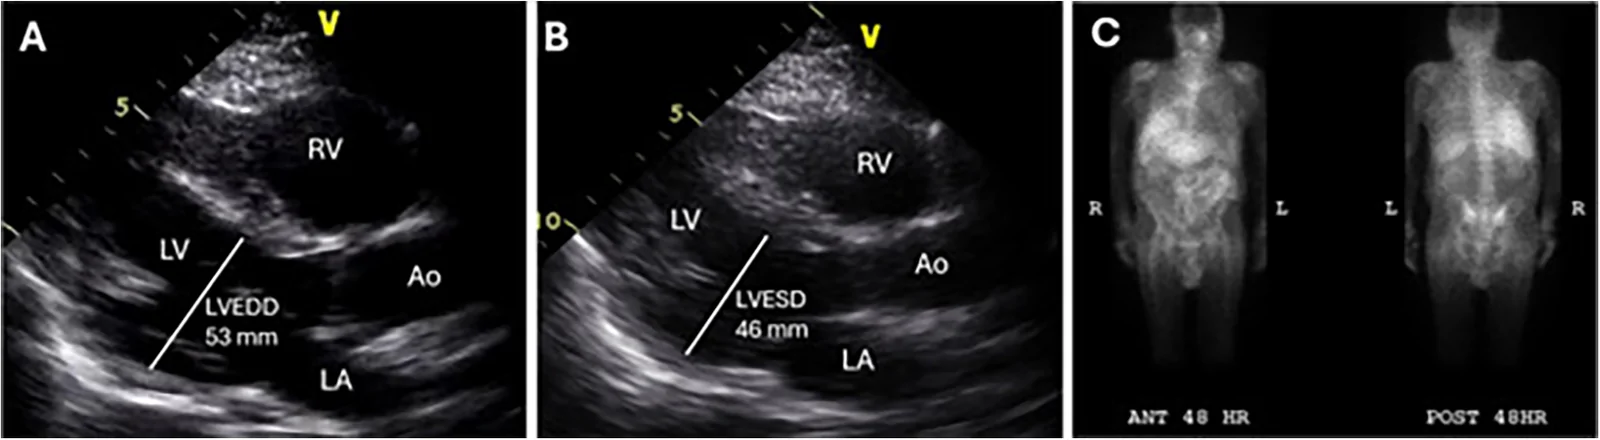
(A,B) transthoracic echocardiography in parasternal long-axis view during diastole (A) and systole (B) demonstrates biventricular systolic dysfunction (left ventricular ejection fraction 32%) with mild right ventricular enlargement and left ventricular dimensions of 53 mm (end-diastolic) and 46 mm (end-systolic). (C) Gallium-67 scintigraphy reveals multiorgan radiotracer uptake, including focal cardiac accumulation, consistent with myocardial infiltration by extranodal NK/T-cell lymphoma.
